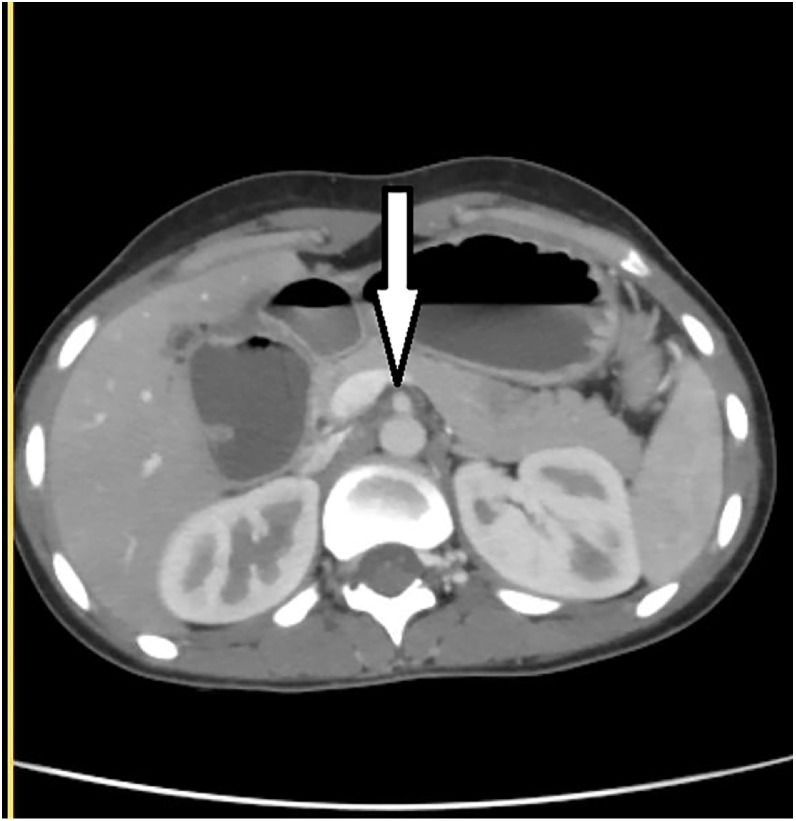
Axial plane; contrast enhanced CT scan of the abdomen (portal venous phase) showing SMA arising from abdominal aorta (arrow).
